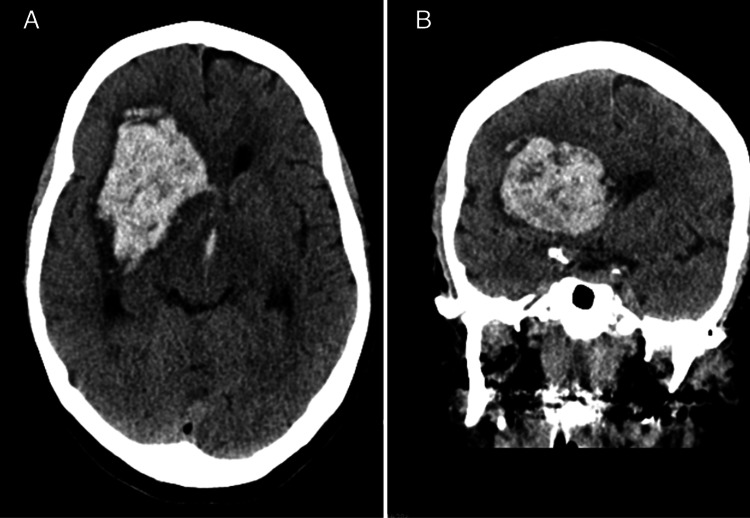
Axial (A) and coronal (B) CT of the head showing right-sided basal ganglia intraparenchymal hemorrhage measuring 8.5 × 4.3 × 3.8 cm with associated brain compression, midline shift, intraventricular extension, and mild perilesional edema.
